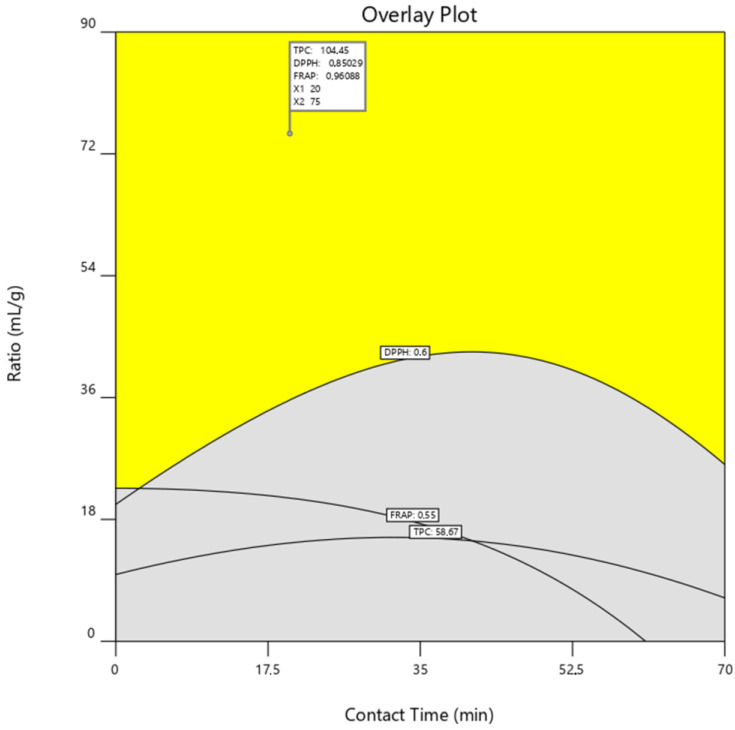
Overlay plot with the optimum point defined for the three evaluated responses as a function of the contact time and solvent-to-solid ratio using UAE methodology. The variables are presented as real values. DPPH, 2,2-diphenyl-1-picrylhydrazyl; FRAP, ferric reducing antioxidant power; TPC, total polyphenol content; UAE, ultrasound-assisted extraction.
